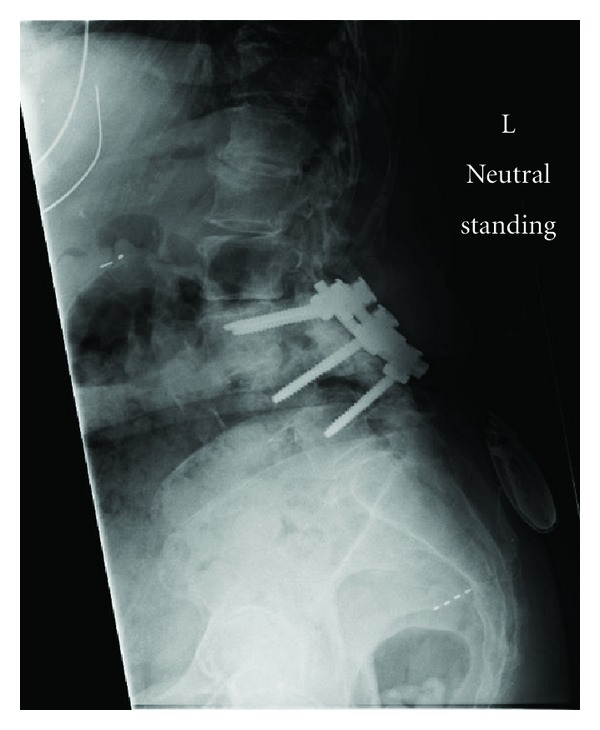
Lateral radiograph of the lumbosacral spine showing prior L3–L5 posterior fusion and instrumentation. However, there is adjacent segment degeneration cephalad to the instrumentation at L2-L3.
